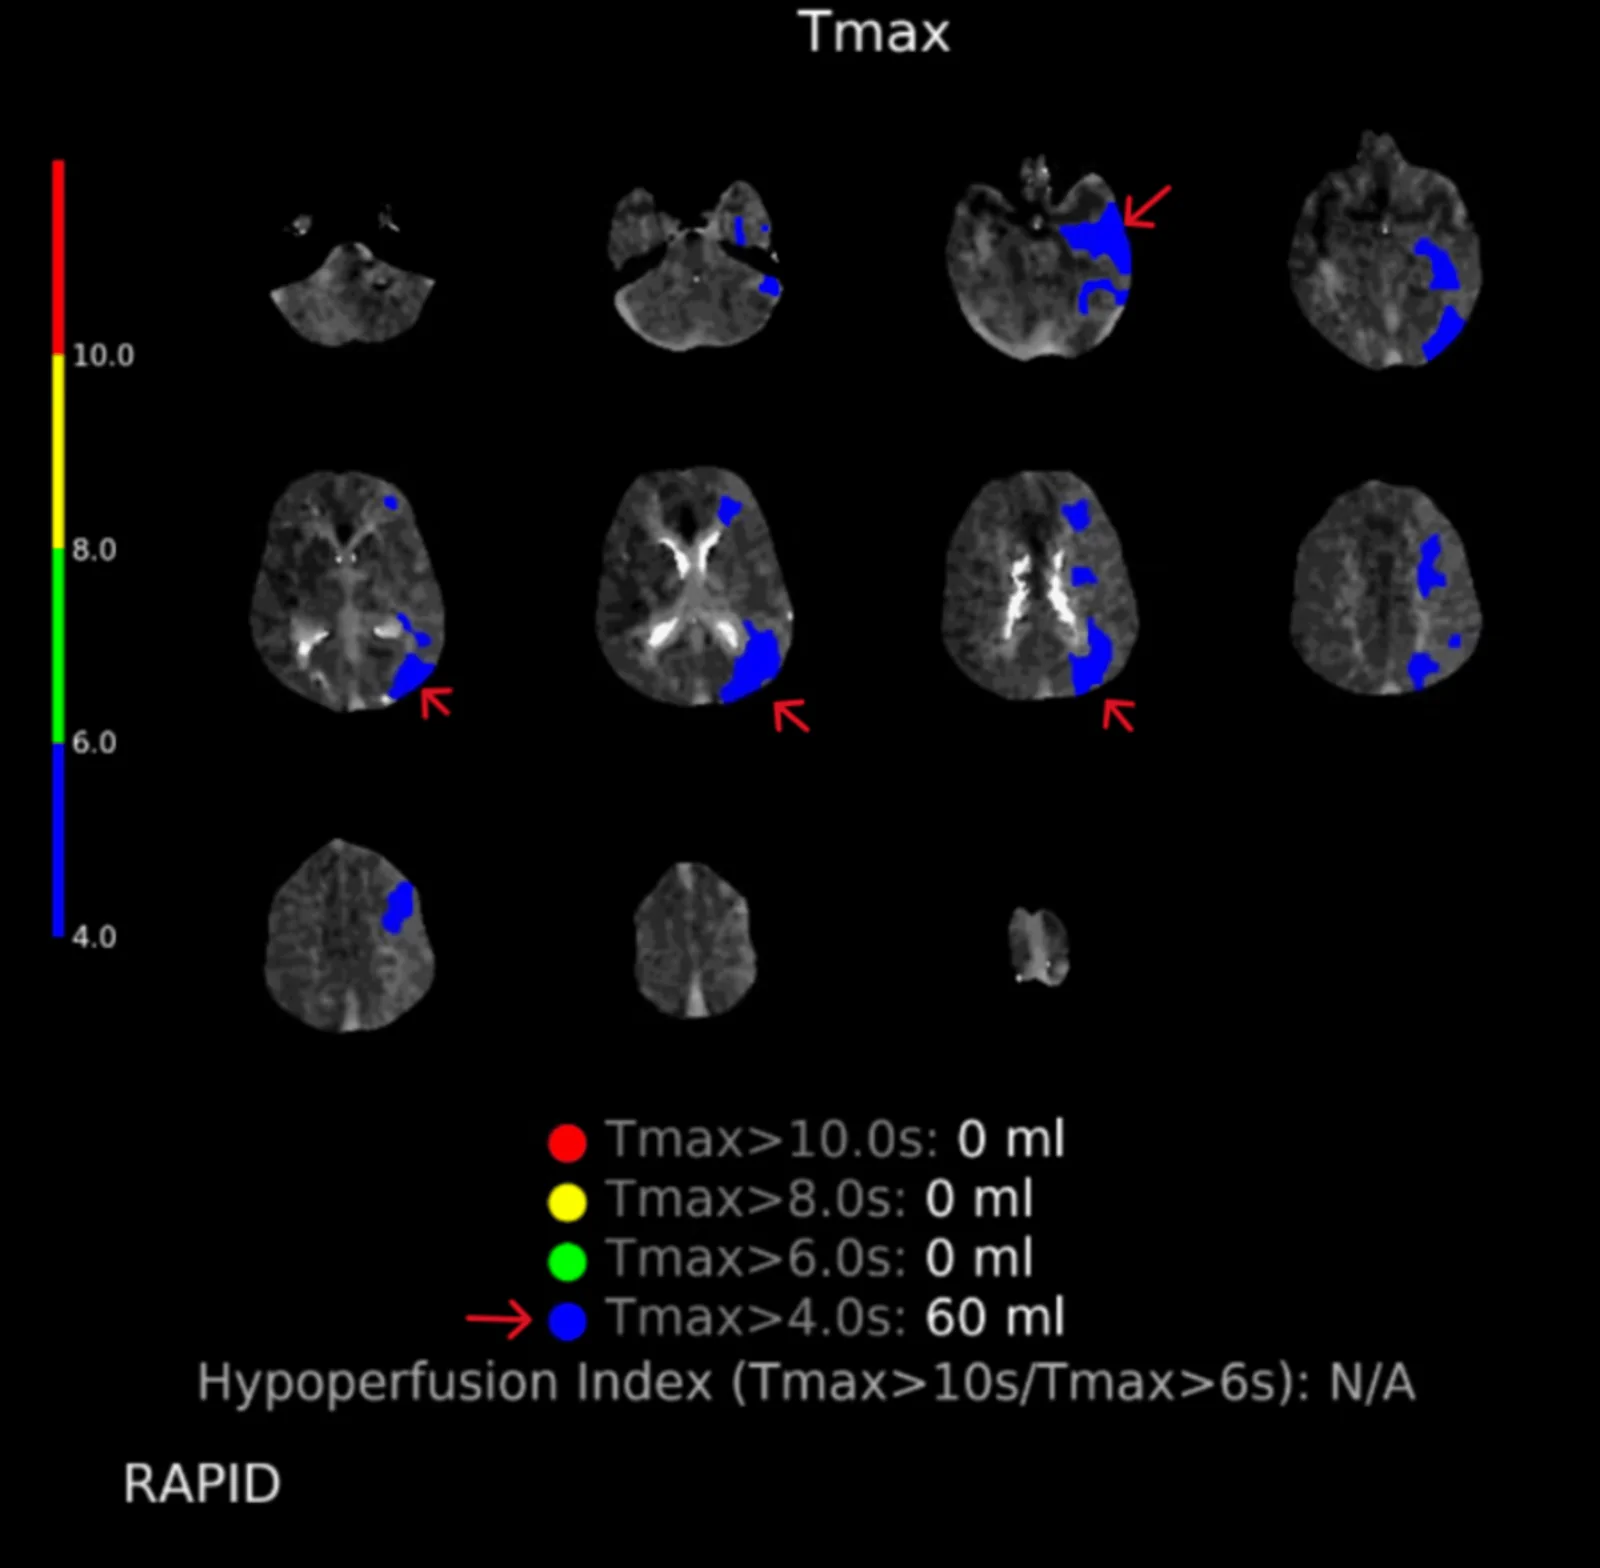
CT perfusion scans demonstrating prolonged contrast transit, with a time-to-maximum (Tmax) >4 seconds involving approximately 60 mL of the left cerebral hemisphere (as indicated by the red arrows).
